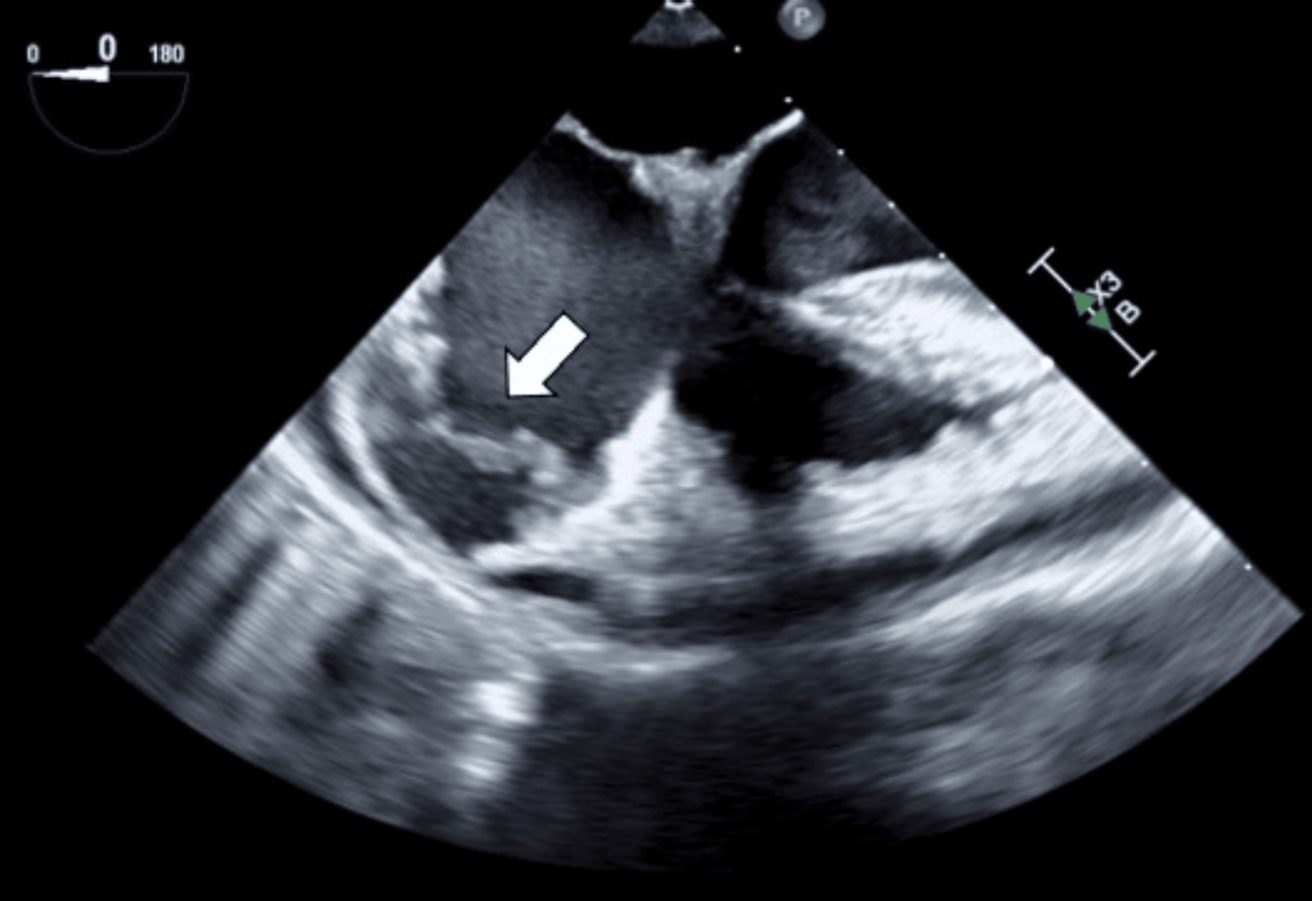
Intraoperative TEE in mid-esophageal four-chamber view during pericardial window surgery revealing a right atrial pseudoaneurysm (arrow) without an obvious tumor In this image, the TEE probe was rotated clockwise from the standard mid-esophageal four-chamber view, primarily highlighting the structures of the right atrium and right ventricle. TEE: transesophageal echocardiography
